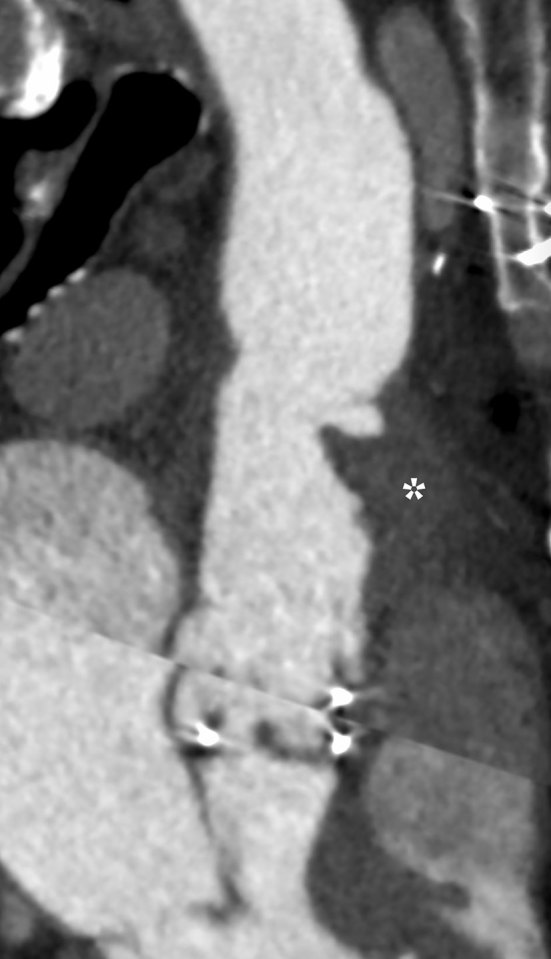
Curved multiplanar reconstruction of CT angiography. The intraluminal vegetation arises from the thickened wall (asterisk) of the graft.
